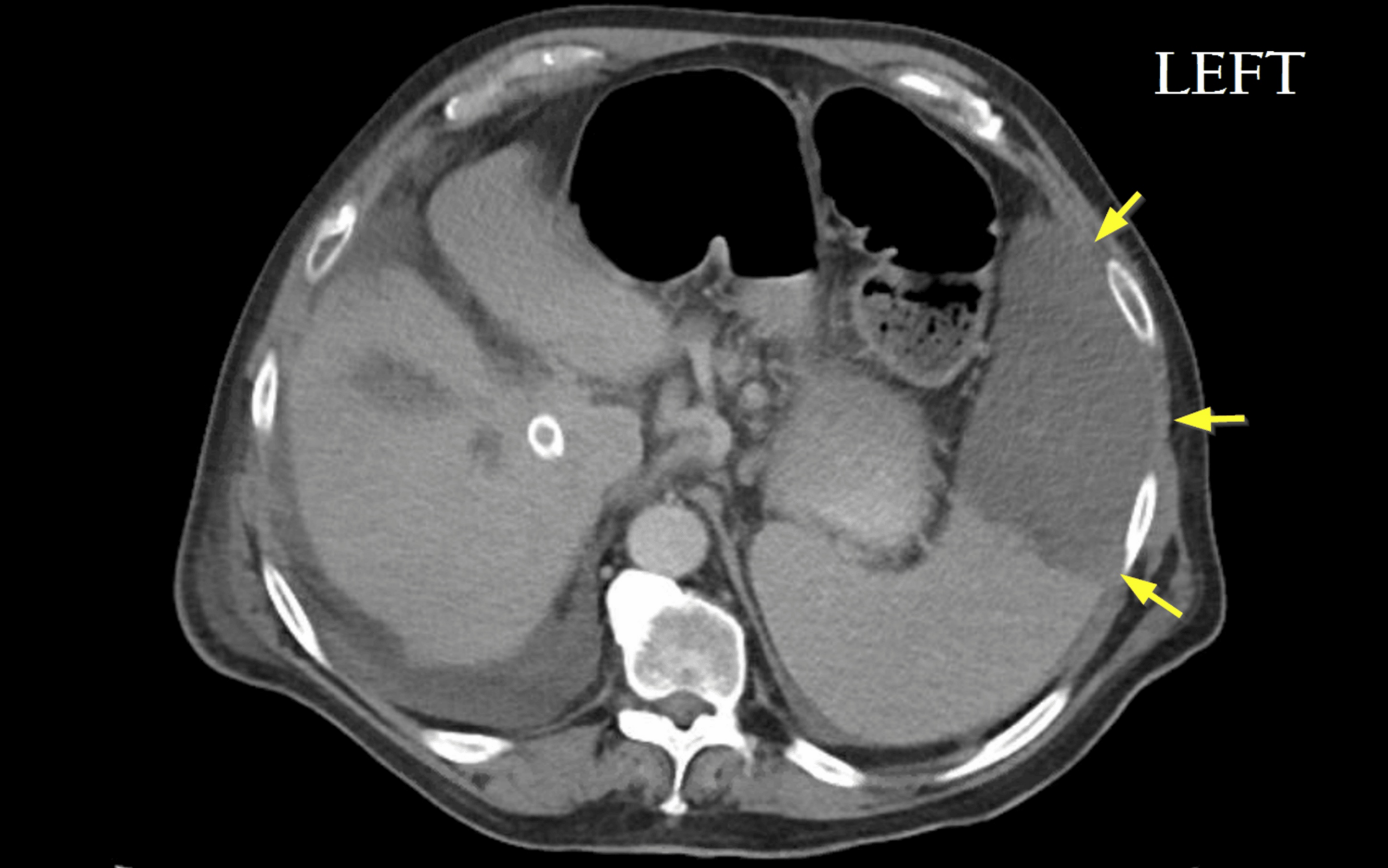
Axial CT image of the spleen after partial splenic embolization. Post-embolization CT demonstrates a reduced splenic size with an area of infarct inferiorly and anteriorly (yellow arrows). Approximate active tissue dimensions post-embolization were 9 × 12 × 14 cm, with a calculated total volume of 907 mL.
